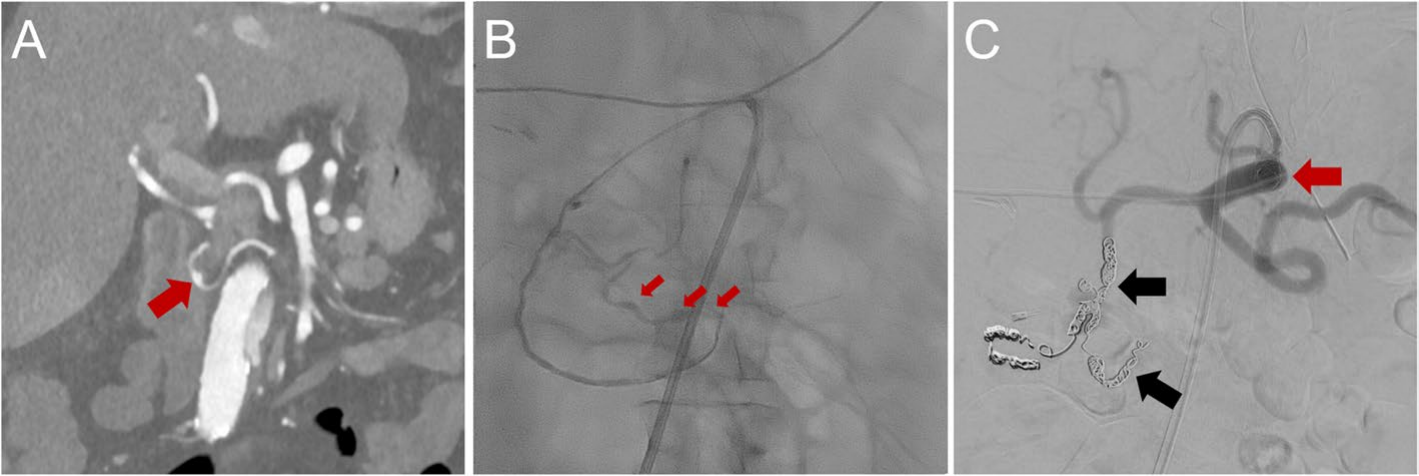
Representative case. CTA and DSA imaging of a 78-year-old female patient, who was referred to our clinic with recurrent duodenal bleeding. A CTA showed a CA stenosis > 50% and a 6 mm × 5 mm × 5 mm superior PDA pseudoaneurysm (red arrow) with an AVR of 2 in a critical position, there was no sign of an active bleeding. B DSA on the following day revealed an active contrast extravasation (red arrows) in position of the diagnosed aneurysm. C Coil embolization (black arrows) of the superior PDA aneurysm in front-and-back-door technique and CA stenting (red arrow) were performed
